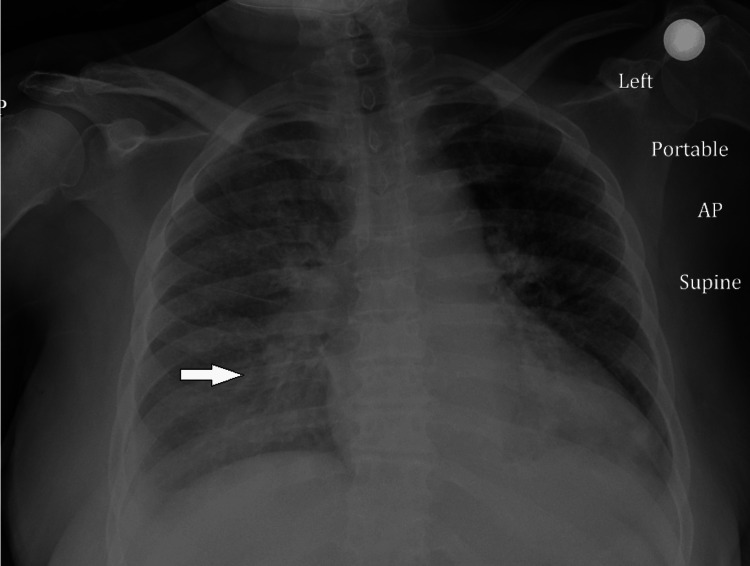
Chest X-ray showing lung infiltrates (white arrow)
